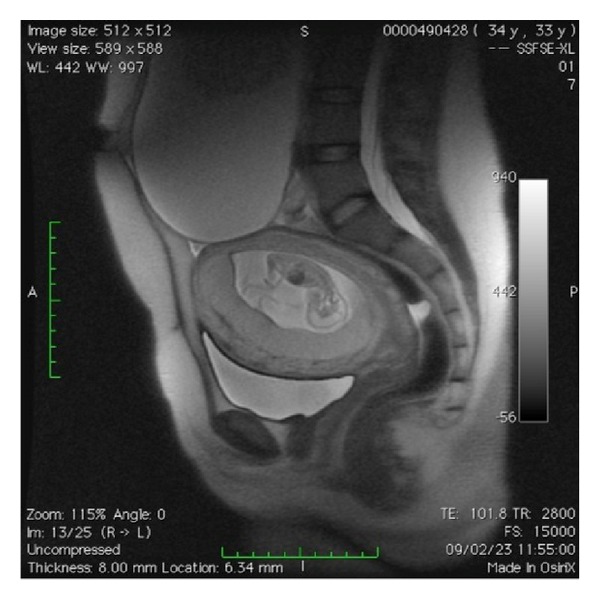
MRI demonstrating the relation between the adnexal mass and the pregnant uterus.
